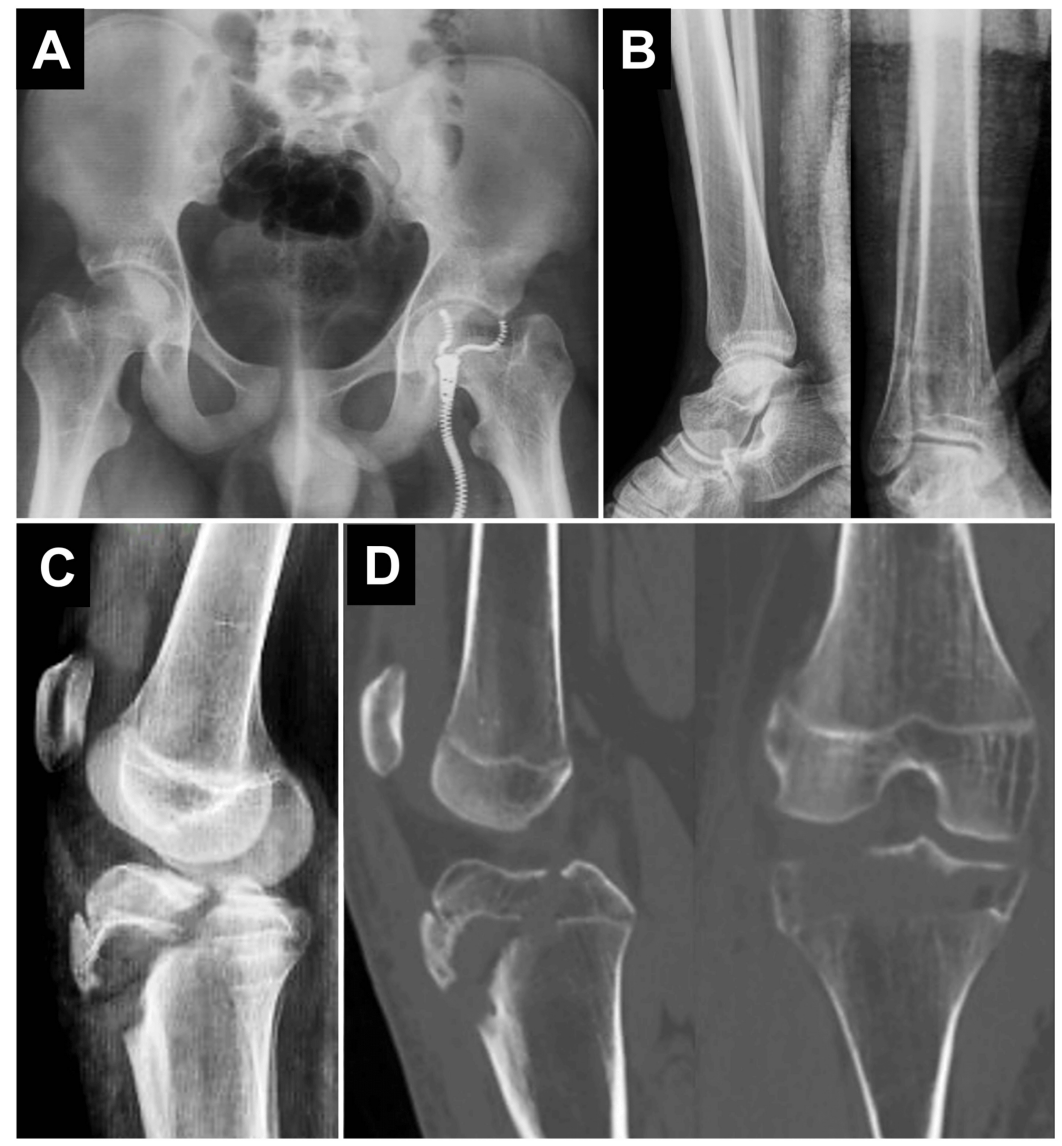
Radiographic overview: historical and preoperative imaging. (A) Anteroposterior pelvic radiograph obtained during previous orthopedic follow-up, demonstrating generalized osteopenia and thin cortical bone, consistent with osteogenesis imperfecta. (B) Prior bilateral ankle radiographs showing metaphyseal modeling and mild deformity. (C) Lateral radiograph of the right knee at presentation, revealing an avulsion fracture of the tibial tuberosity. (D) Coronal and sagittal computed tomography images confirming a Salter-Harris type III (Ogden IIIA) fracture involving the proximal tibial epiphysis with posterior extension into the articular surface, without evidence of comminution or joint incongruity.
